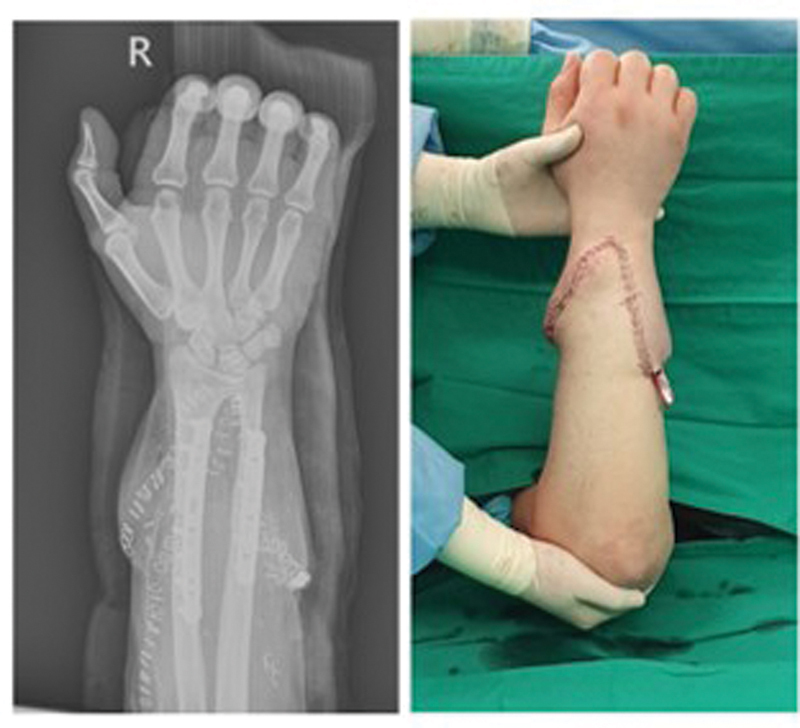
Immediate postoperative gross photo and X-ray. The donor bone was resected 1.7 cm distal to the proximal border of the pronator quadratus muscle to adjust the bone thickness at the bone fixation level. The bone thickness was well matched at the bone fixation level.
